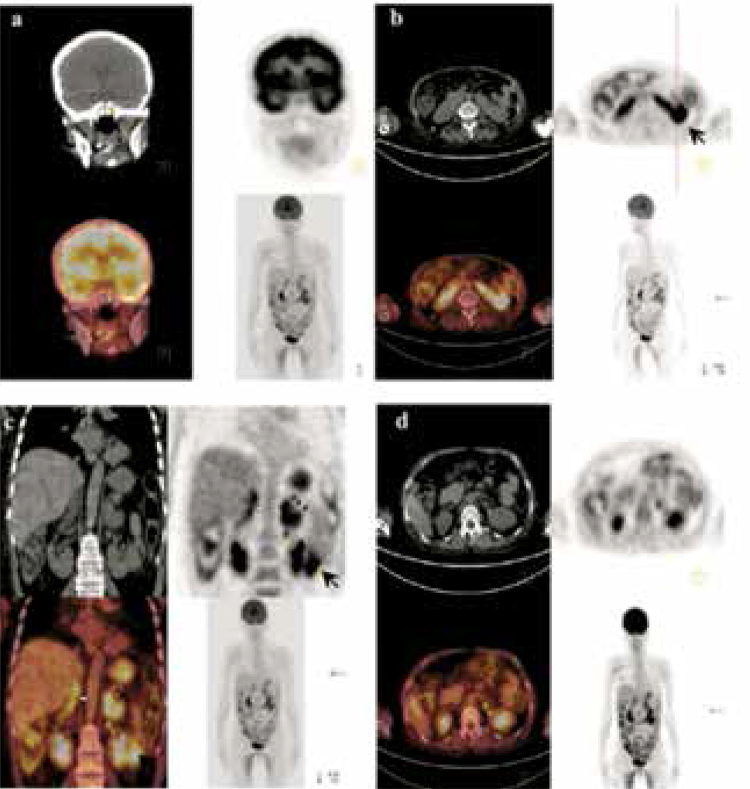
PET/CT scan. (A) Shows a mild focal uptake in the pituitary gland region. (B) Demonstrates horseshoe kidney deformity and intense increased activity in the lower pole of the left kidney (thick black arrow). (C, D) Show heterogeneous increased uptake in the right adrenal gland (thick white arrow), intense increased uptake in the left adrenal gland (black arrowheads), and a focal increased uptake in a lymph node located in the inferior adjacent to the left adrenal gland (thin yellow arrows).
